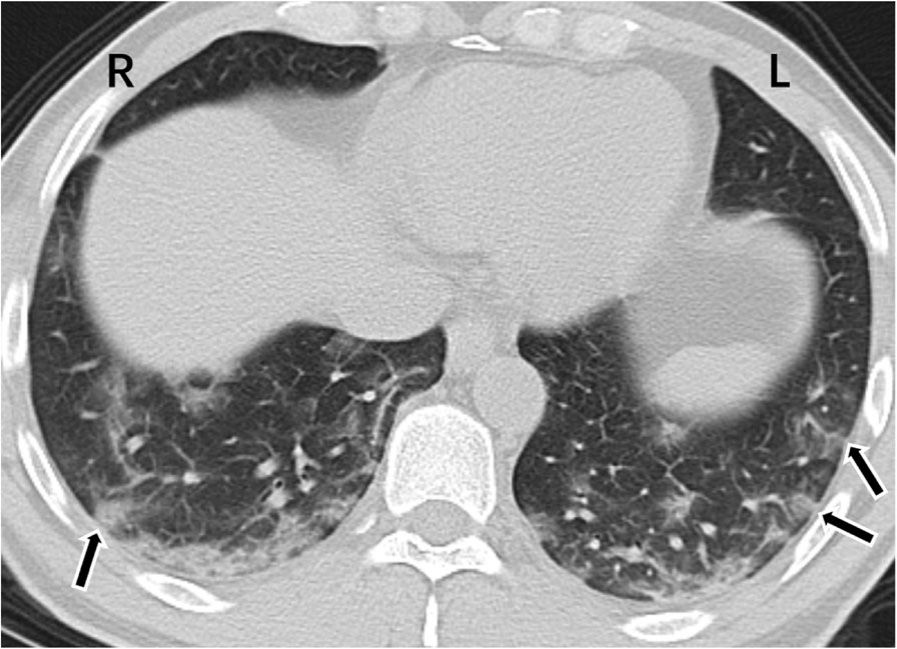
CT image of another 46-year-old male patient showing multiple areas of small patchy GGO on the lateral and posterior basal segments of both lower lobes, with many lesions immediately adjacent to the subpleural region. There is visible localized pleural thickening (long black arrow), but no obvious pleural effusion is observed
